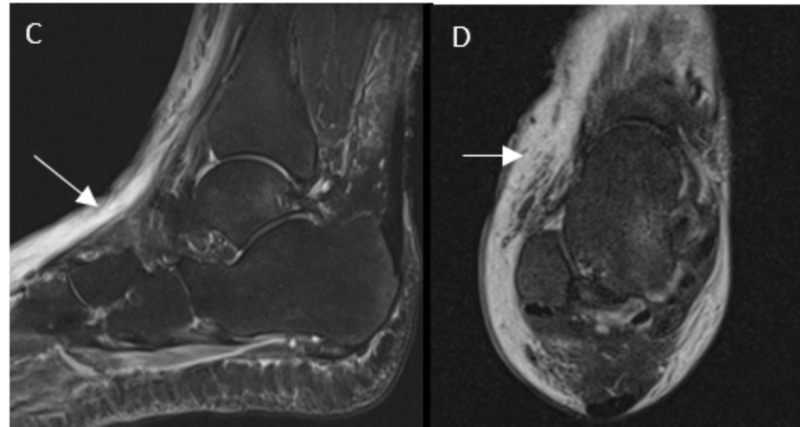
MRI scan of the right foot C: Sagittal and D: axial images showing dorsal ankle and soft tissue swelling with edema (arrow).
